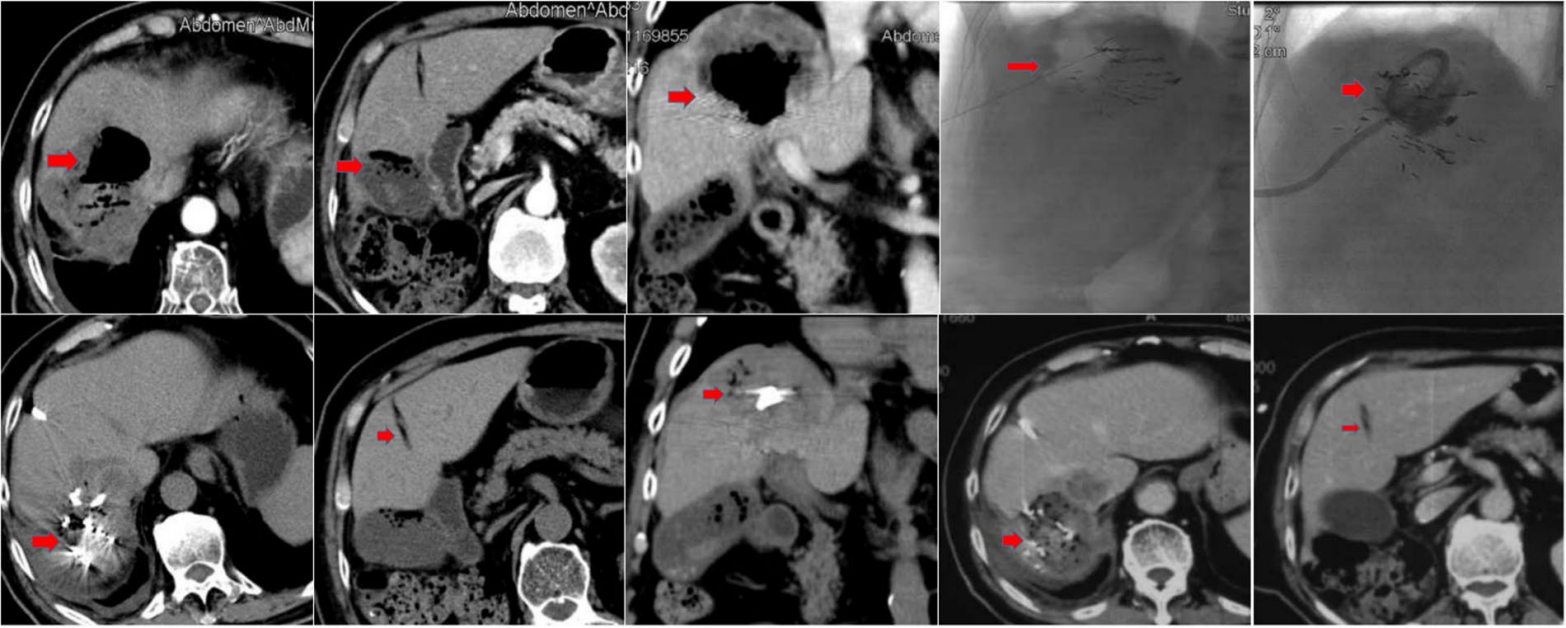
CT images of a 61-year-old male patient diagnosed with HCC. Four days after TACE (doxorubicin 50 mg and CalliSpheres of 100–300 µm, April 20, 2017), the patient suffered from an acute infection with biloma. The patient achieved a stable clinical condition after external drainage and antibiotic therapy. The top left CT images show biloma formation (April 24, 2017). The top right CT images show external drainage of biloma (April 24, 2017). The bottom left CT images show complete disappearance of the biloma 1 day after treatment (April 25, 207). The bottom right CT images show tumor necrosis with no biloma (July 4, 2017). The patient was in stable condition.
